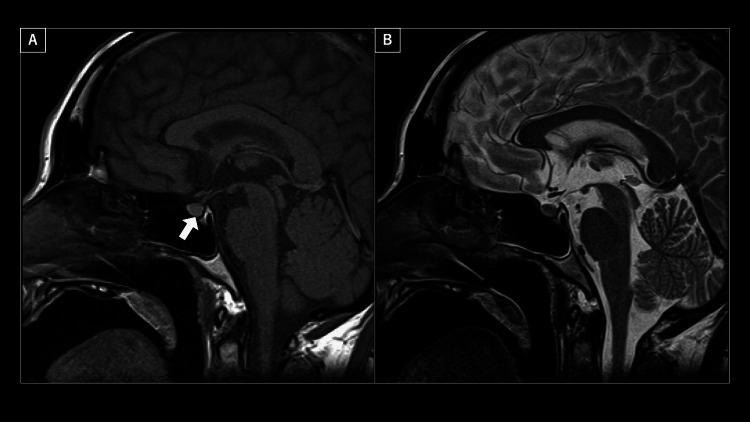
Sagittal cross-sectional MRI image of the head (A) T1-weighted image (arrow indicates loss of high signal in the posterior pituitary gland). (B) T2-weighted image.
